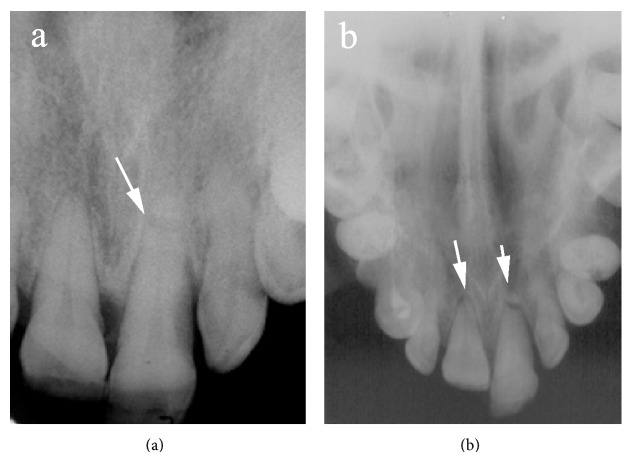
The radiographic view of the patient before treatment. (a) Arrow marks the horizontal root fracture. (b) Arrow marks the enlarged periodontal space of right and left maxillary teeth.
